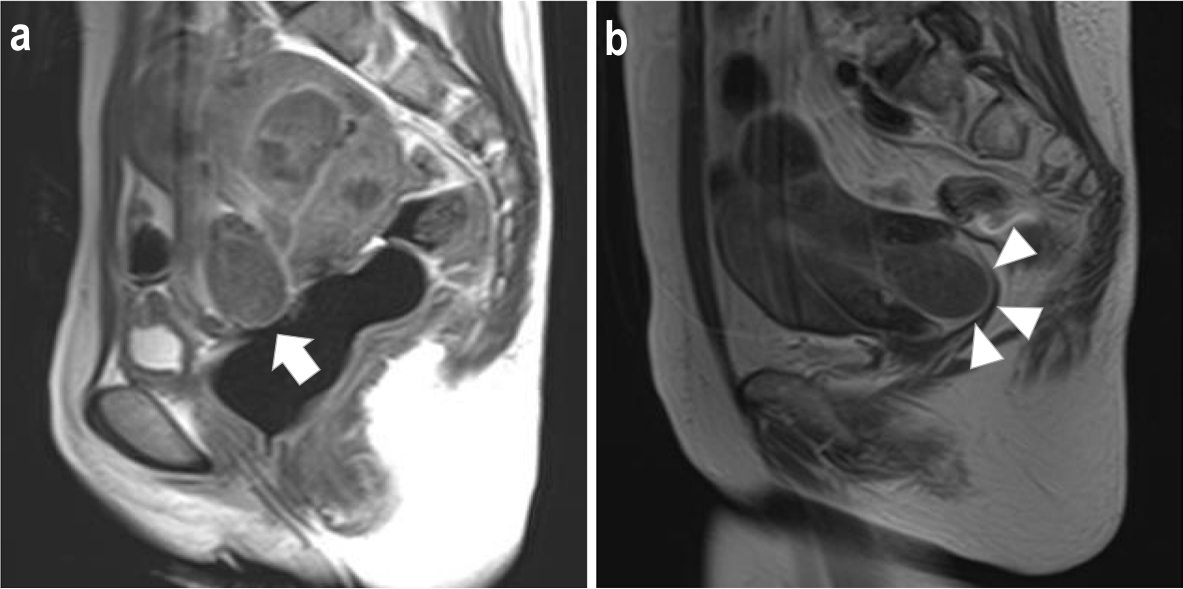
The two patients who developed severe hemorrhage a. Patient 1 (post-treatment) T2-weighted MRI sagittal view. Arrow: The pedunculated myoma prolapsing through the cervix (33 × 27 × 24 mm) b. Patient 2 (pre-treatment) T2-weighted MRI sagittal view. Arrowhead: The pedunculated myoma prolapsing through the cervix (61 × 43 × 31 mm).
